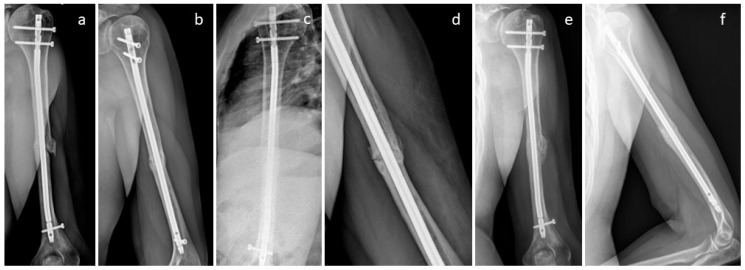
A non-union in the humerus shaft of a patient. (a,b): Presurgical X-rays. (c,d): The nail was left in, but the non-union was bridged with three Shark Screws^®^, as shown in the post-surgical X-rays; union was recorded after seven weeks. (e,f): Eight months after revision, the Shark Screws^®^ were remodelled into the host bone and are no longer visible.
